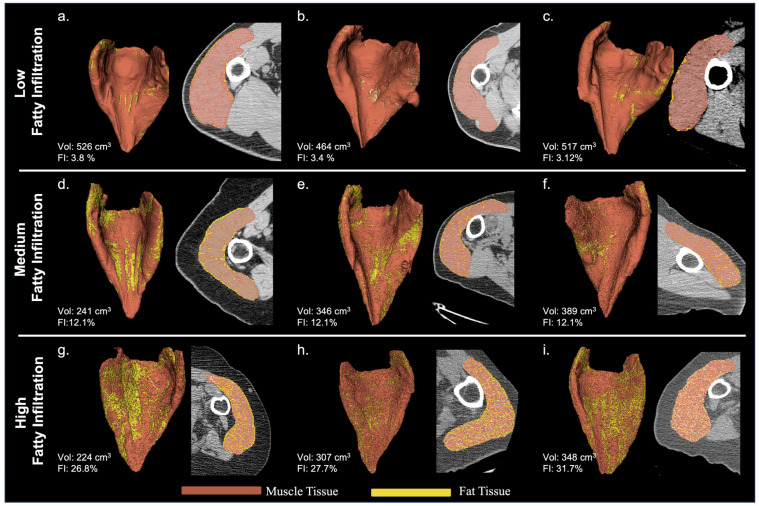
Representative Deltoid Muscle with Low ((a–c): top row), Medium ((d–f): middle row), and High ((g–i): bottom row) Levels of Fatty Infiltration for 3D Volumes Reconstructed from BONE Kernel CT Images.
